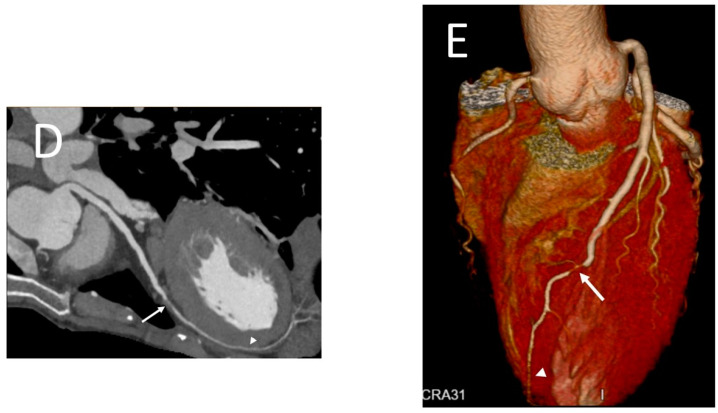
Series shows catheter angiogram (A) which shows moderate stenosis of the mid left anterior descending coronary artery (LAD; white arrow) and occlusion of the distal LAD (white arrowhead) with distal collaterals (black arrow). No other significant stenosis in rest of the coronary arteries (not shown) seen, findings which are consistent with SCAD. Cardiac MR (B,C) performed later revealed resting perfusion defect (B) in the apical inferior wall (arrow) with corresponding subendocardial (near 75%) late gadolinium enhancement (arrowhead; C) consistent with an infarct in the distribution of the left anterior descending coronary artery. Three days after discharge and cardiac MR, the patient presented again with band-like c lower chest pain and coronary CT angiogram (D,E) was performed. Curved planar reformat (D) and volume rendered (E) images now revealed focal severe stenosis (white arrows) in the mid LAD with recanalization of the distal segment (arrowhead) compared to the catheter angiogram performed five days prior.
